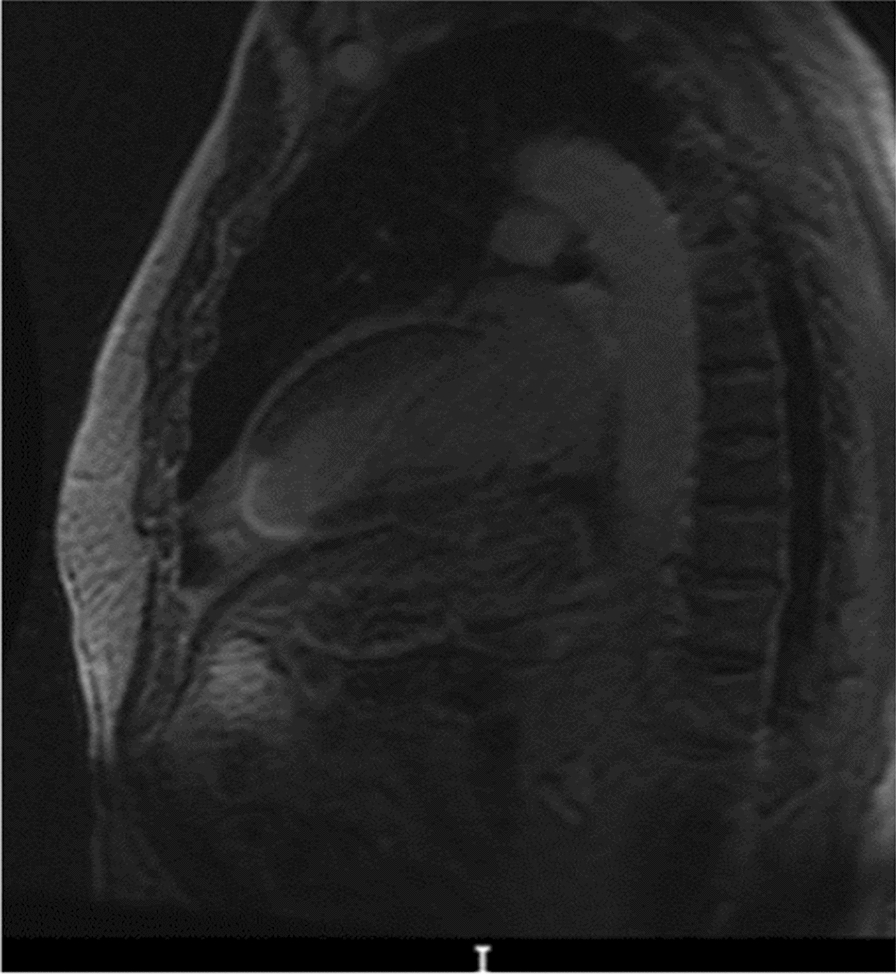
MRI (sagittal plane) showing cardiac fibrosis
